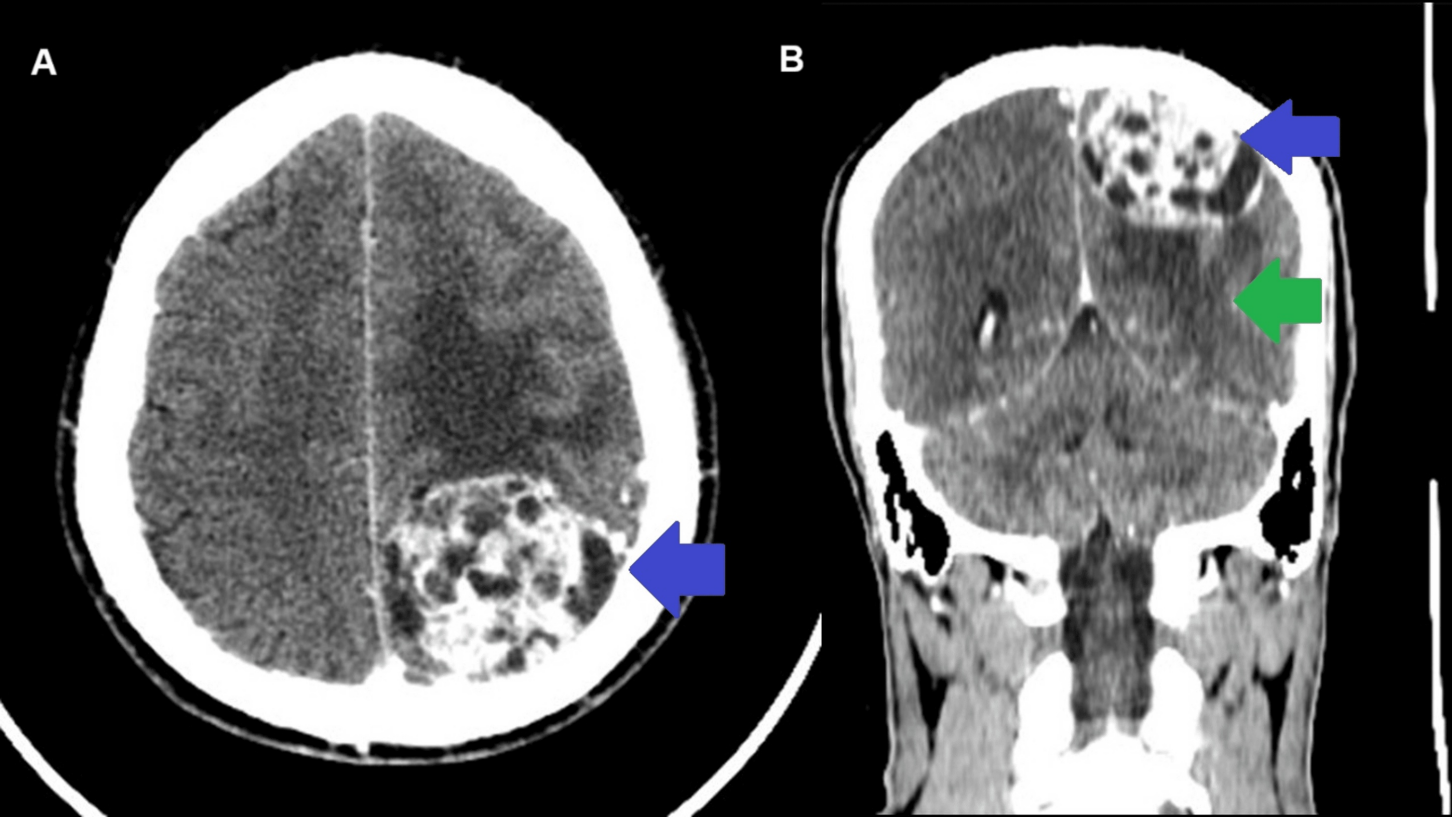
Contrast-enhanced head CT scans. (A) Axial and (B) coronal views showing a 44 × 44 mm heterogeneous hypodense parietal lesion with intense enhancement (blue arrows), causing mass effect with sulcal effacement, perilesional edema, and compression of the left lateral ventricle (green arrow).
